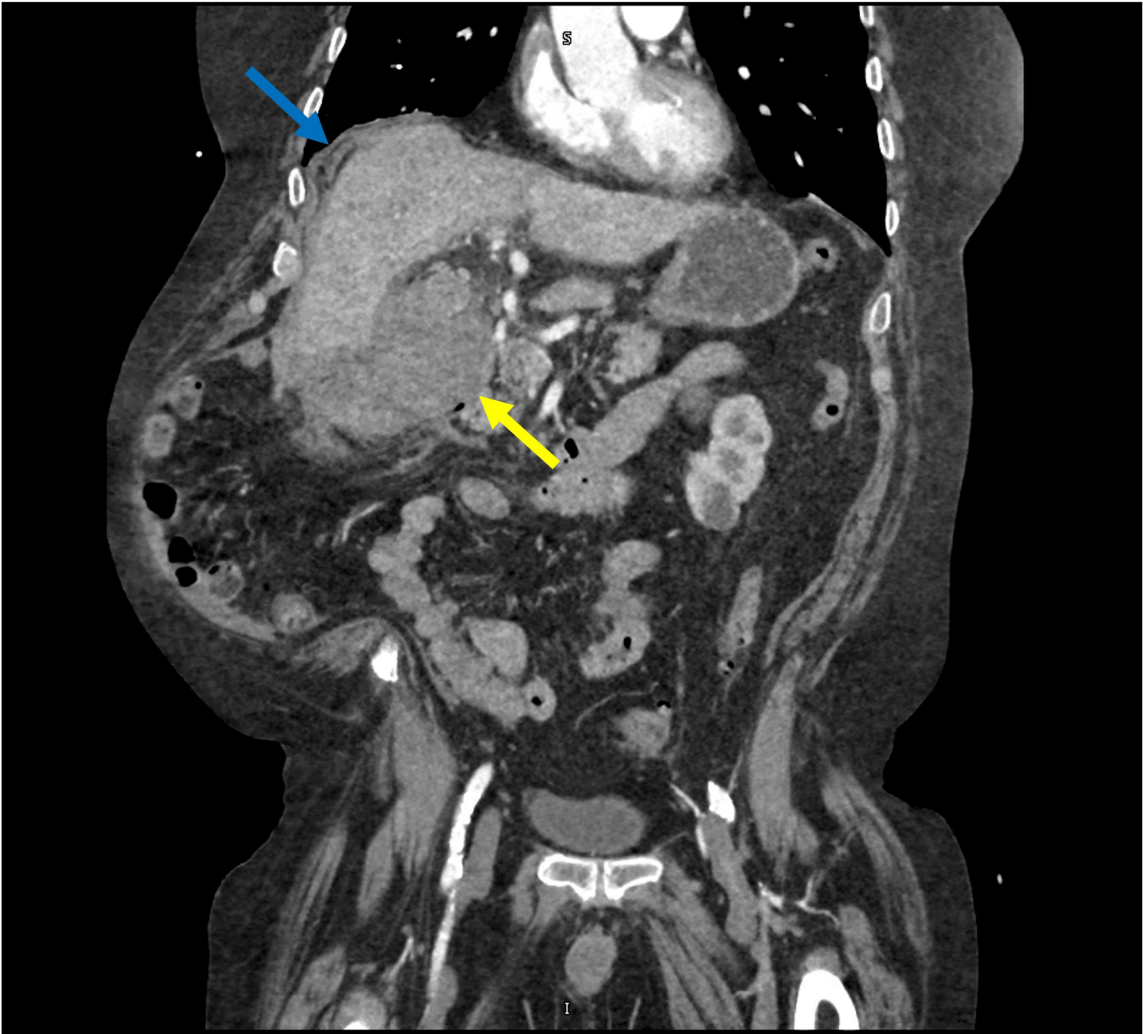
Coronal abdominal and pelvic CT scan showing significant gallbladder distention (yellow arrow) and subhepatic fluid (blue arrow).
